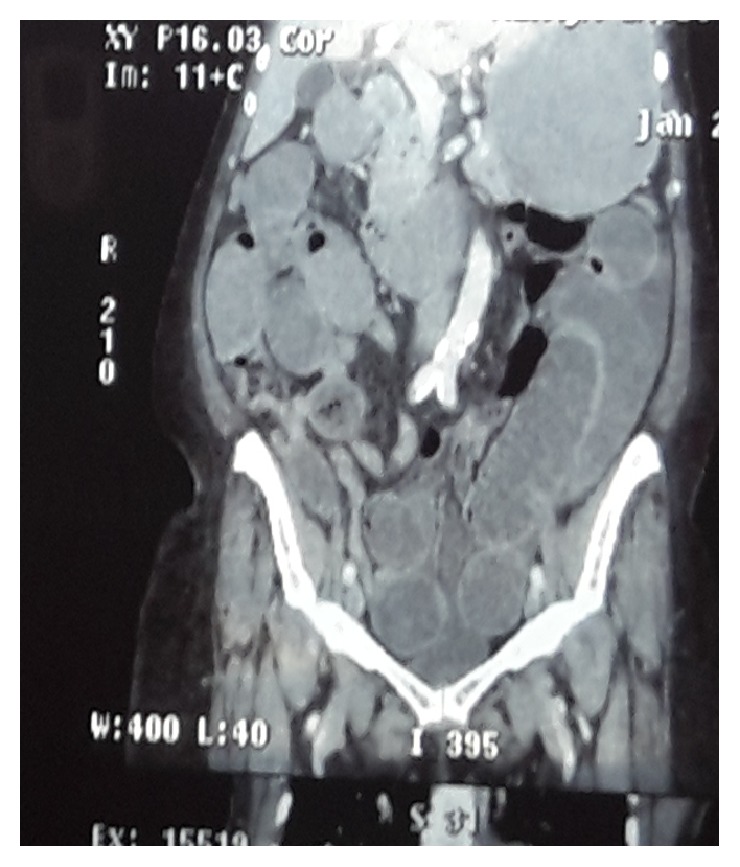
Coronal view of the computed tomography (CT) of the abdomen showing grossly dilated thickened small bowel loops up to the region of right iliac fossa.
